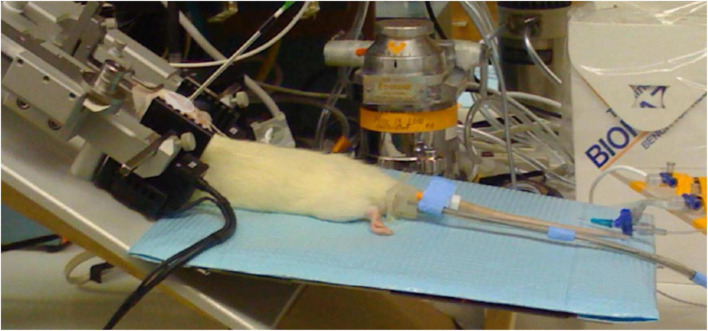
Caloric ear bars in place, laser Doppler probe affixed; head flexed upward by 30 degrees, support pad tilted slightly upward.
